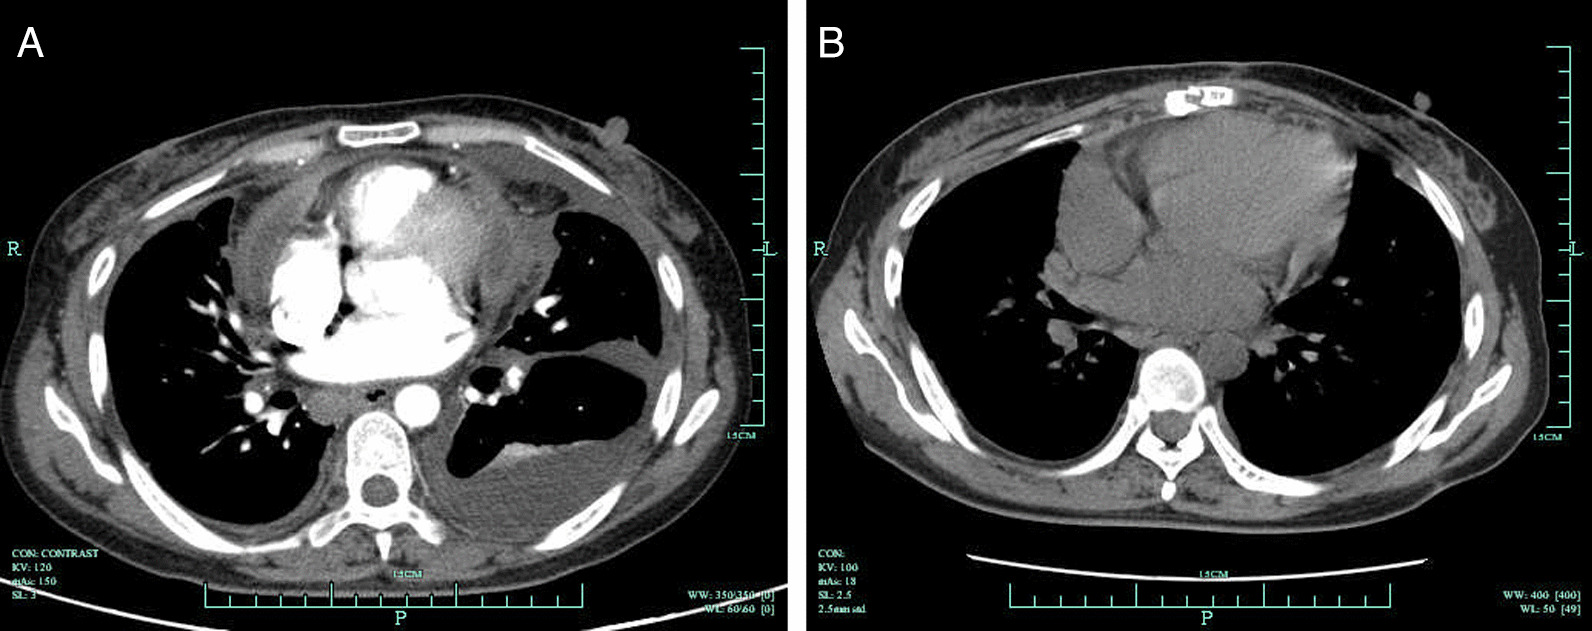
A The preoperative CT picture of a patient with constrictive tuberculous pericarditis. B The CT picture of the same patient at 2 month postoperatively
